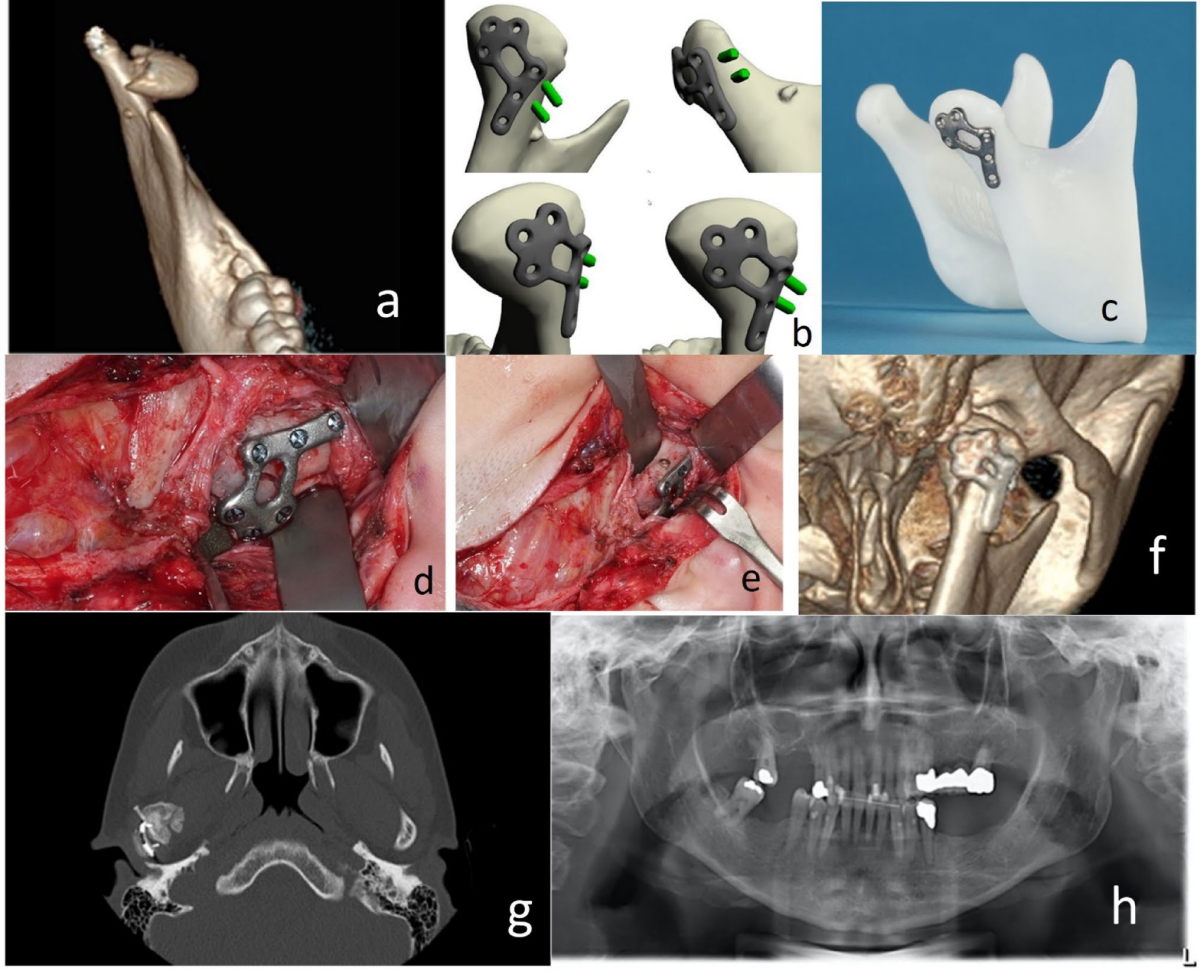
Case 2: Preoperative 3D CT-Scan – right mandibular CHF (a). VSP – mirrored condyle with P-shaped PSD. Outer portion of two screws for lateral fixation in green (b). CAM – PSD on STL model (c). Intraoperatively – fixation of the P-shaped PSD after CHF reduction (d). Intraoperatively – head of single 15 mm long small fragment screw in ramus fracture end (e). Postoperative 3D CT-Scan – combined PSD and LSFPSO reconstruction (f). CT-Scan 7 months postoperatively – PSD with loosening of its fixation screws (g)
